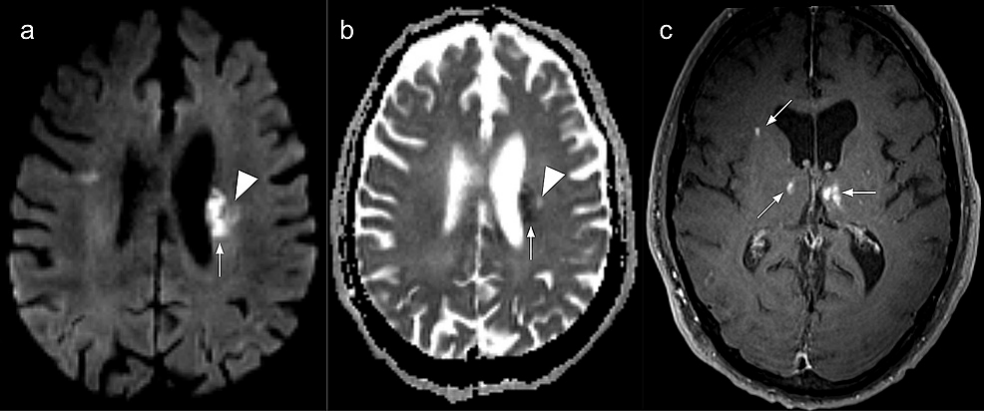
Brain magnetic resonance imaging Multiple and bilateral ischemic lesions in various evolution stages. (a) Axial diffusion-weighted image and (b)  apparent diffusion coefficient (ADC) map showing an acute periventricular ischemic lesion (arrows). A chronic lesion is also seen as a facilitated diffusion area (arrowheads); (c) Axial contrast-enhanced T1-weighted image depicting enhancing subacute ischemic in both thalami and the right corona radiata.
